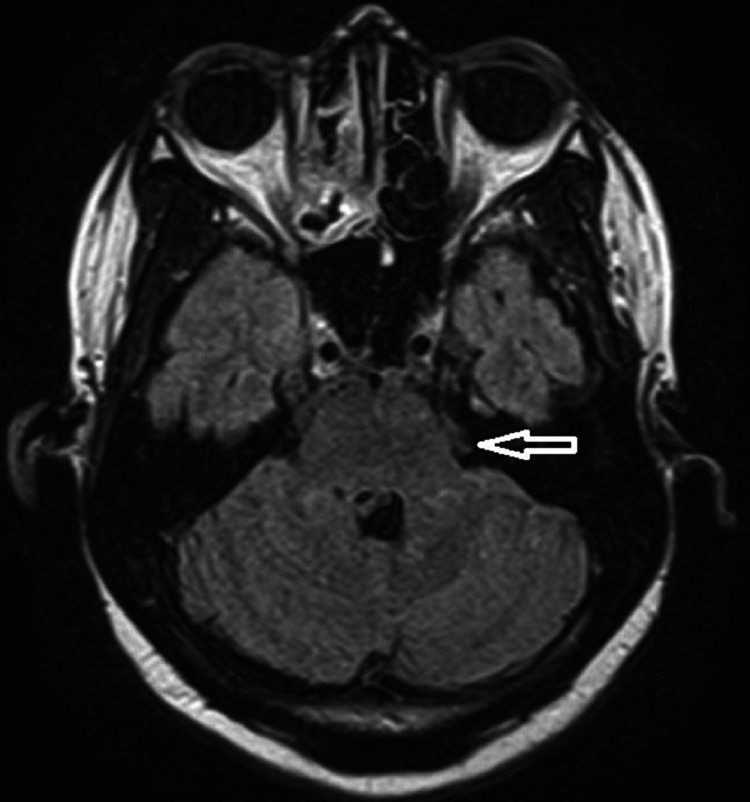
FLAIR imaging. The arrow shows the resolution of the hyperintense lesion in the left brachium pontis. FLAIR: Fluid-attenuated inversion recovery
